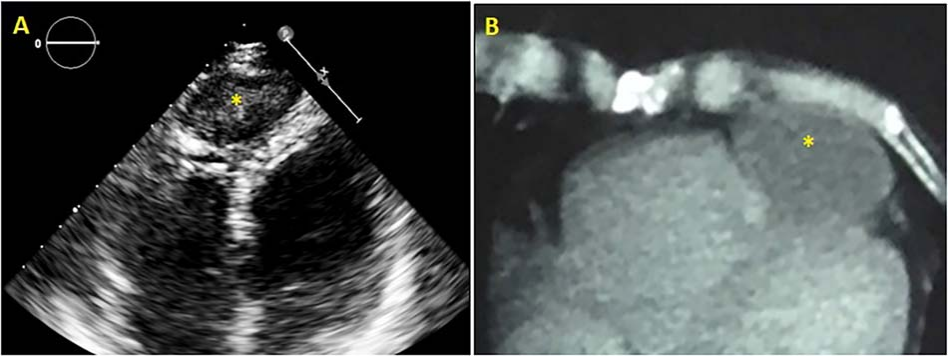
TTE apical four chamber view (A) and CT scan axial view (B) showing intrapericardial hydatid cyst’s evolution to the class CE4/5 (WHO) 2 years after discharge.
